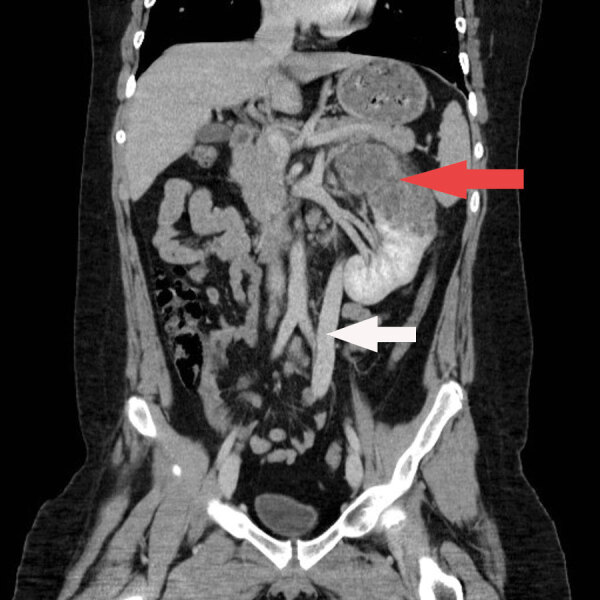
Coronal contrast enhanced CT (computed tomography) of the abdomen of a child with disseminated *Echinococcus multilocularis* infection without liver involvement, Canada, 2018. There is a large irregular hypodense left renal lesion (red arrow). A large porto-systemic shunt is partially visualized (white arrow).
